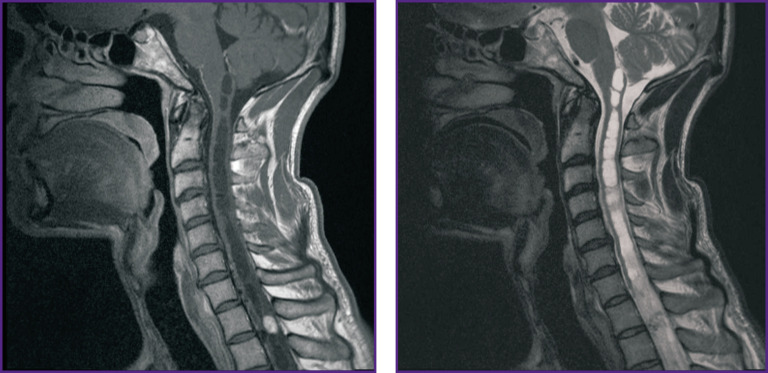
MRI of a patient with intramedullary hemangioblastoma at Th_1_–Th_2_ level (source: authors)
